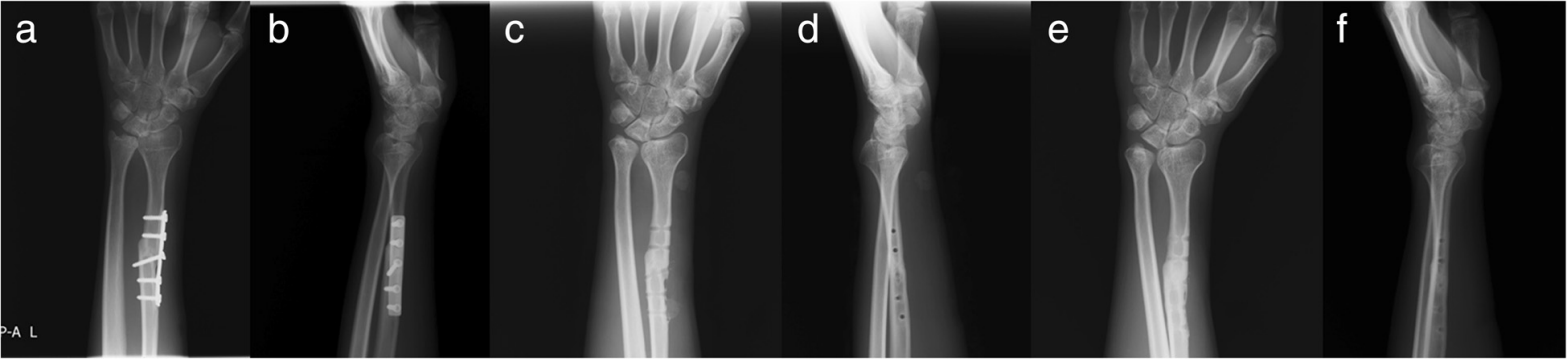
Anteroposterior and lateral X-ray images taken 18 months after wrist reconstruction, before and after the removal of internal fixation (a-d), and images taken 30 months after wrist reconstruction (e, f)
